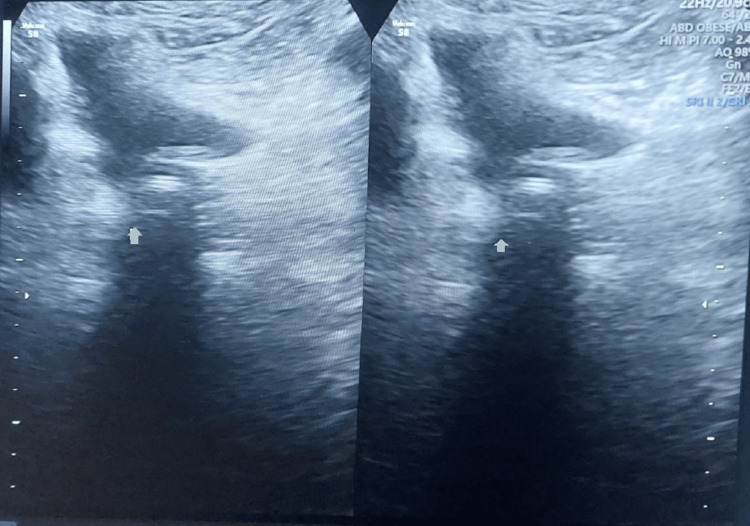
Post-delivery transvaginal sonography showing mesh in proper position We have done a post-delivery transvaginal sonography, which shows mesh is in the proper place, visible as an echogenic linear shadow
